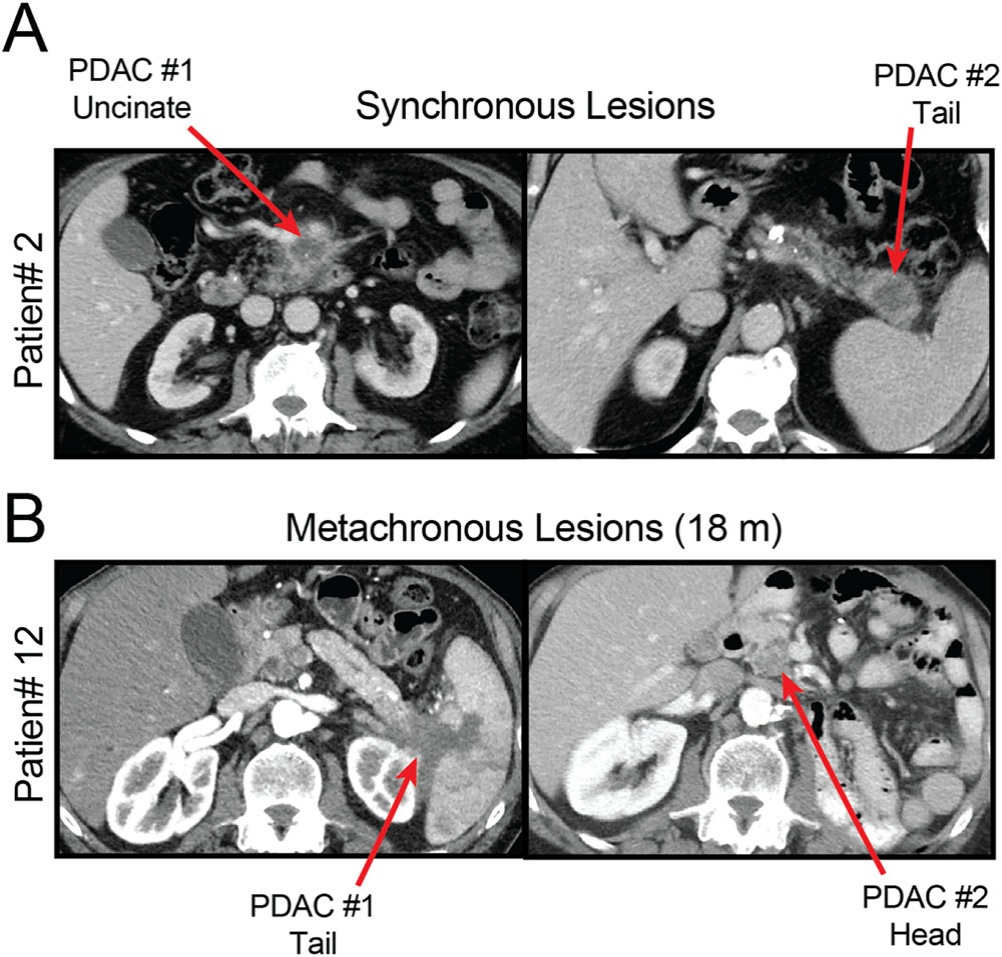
Synchronous and metachronous occurring PDAC. Representative computed tomography (CT) scans demonstrating A synchronously (patient 2) or B metachronously (patient 12; 18-month interval) occurring PDAC without radiographic connection.
